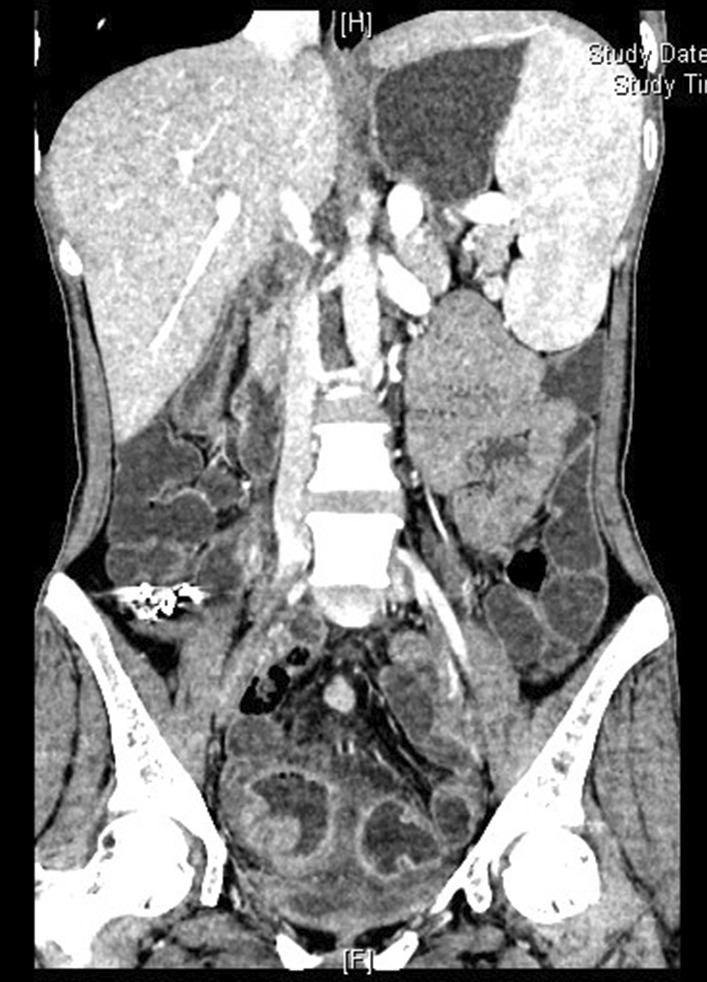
The small-intestine stimulation enhanced computer tomography (CT) examination revealed an unevenly thickened and strengthened wall of the sigmoid colon, splenomegaly, and portal hypertension
